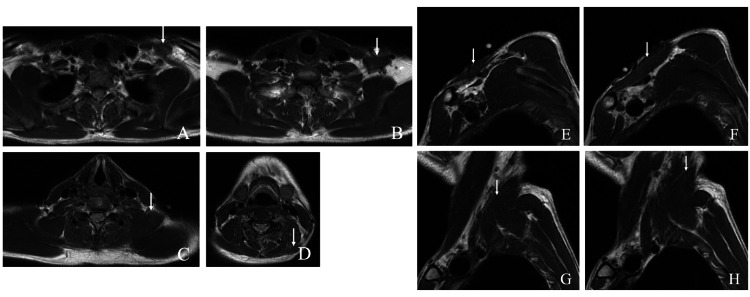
Magnetic resonance images of the neck T1-weighted magnetic resonance images of the neck (A, B, C, D, E, F, G, H) show the anomalous muscle. It originates from the nuchal ligament and stops in the middle one-third of the clavicular head side (white arrow).
